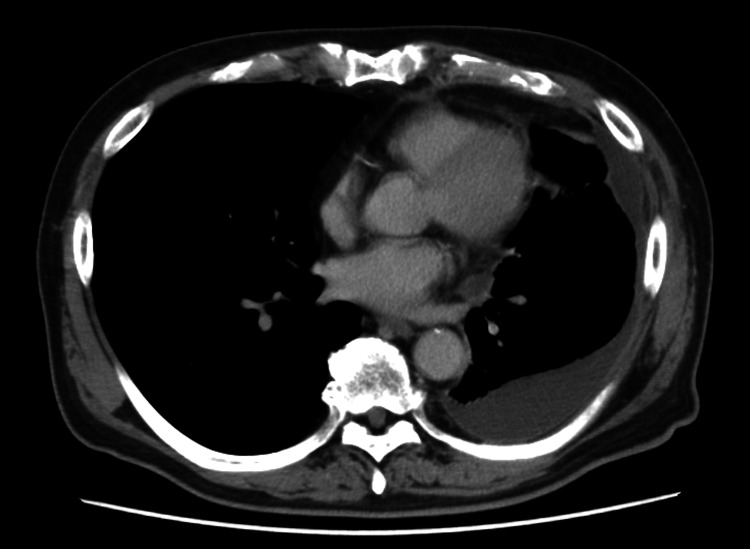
Contrast-enhanced chest computed tomography performed at presentation revealed left pleural effusion.
